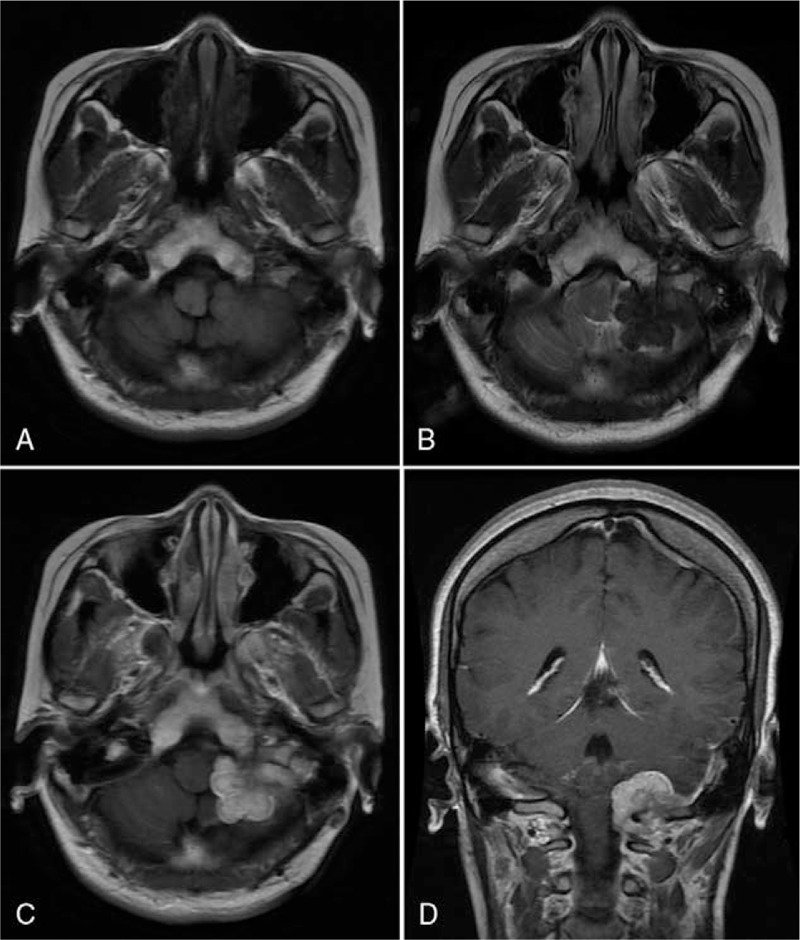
Contrast-enhanced magnetic resonance imaging (MRI) of the brain showed a well-defined, lobulated, homogenous mass in the left foramen magnum (A–D). The lesion was isointense on T1-weighted images (A), hypointense on T2-weighted images (B), and showed homogeneously obvious enhancement following the intravenous administration of gadolinium (C–D). It was dural based and extending inferiorly along the spinal dura up to the cervical spinal canal. The brain stem was compressed and deflected to the right side (A–D).
